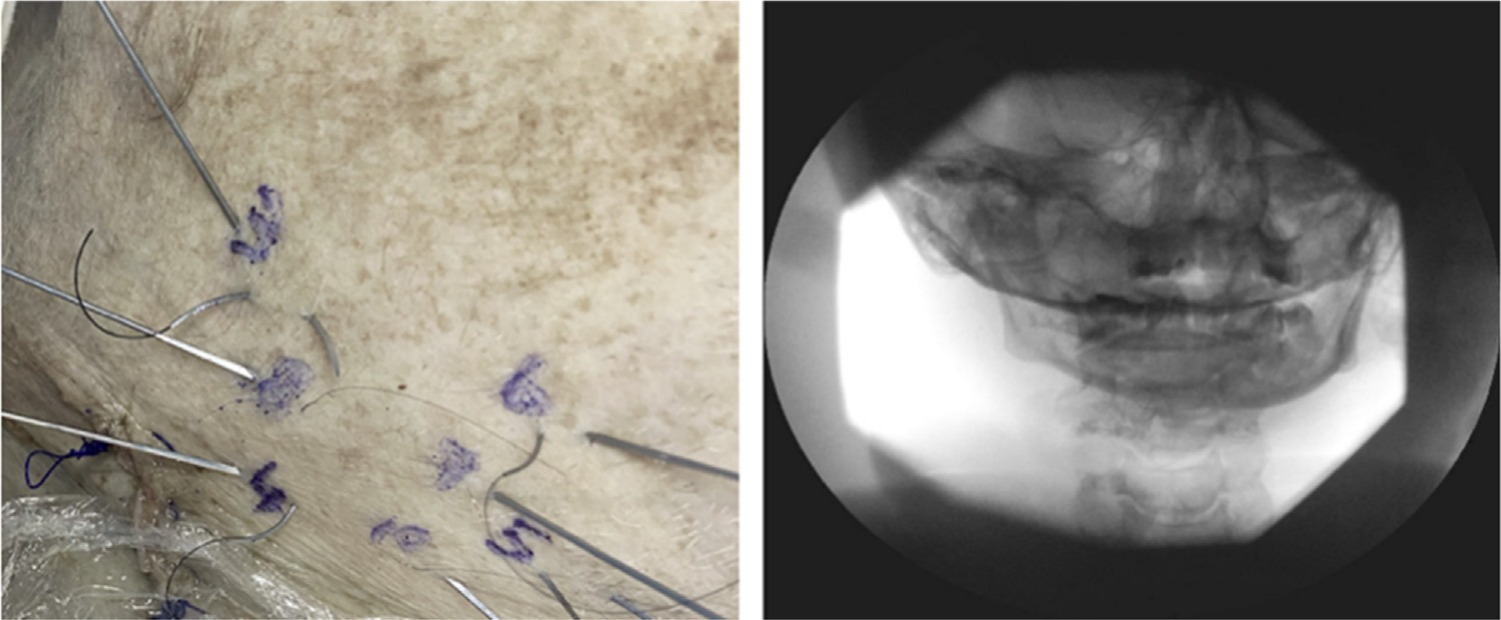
Mandible images/radiograph for needle identification.
